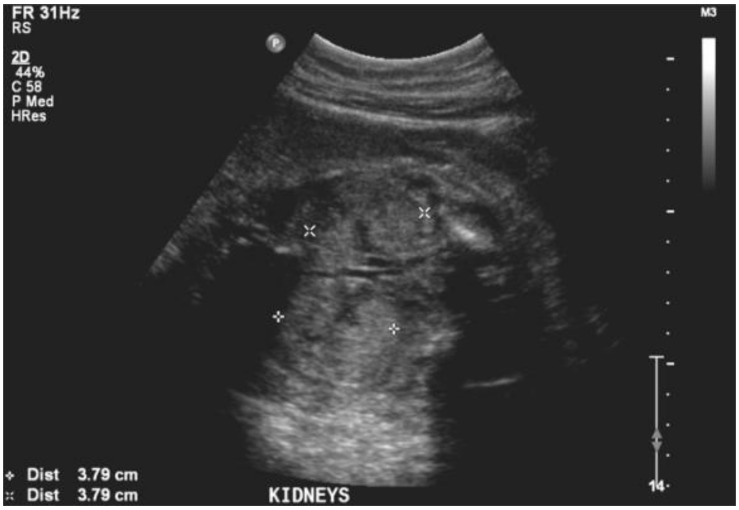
Ultrasound image of fetal kidneys at 27 weeks of gestation.
